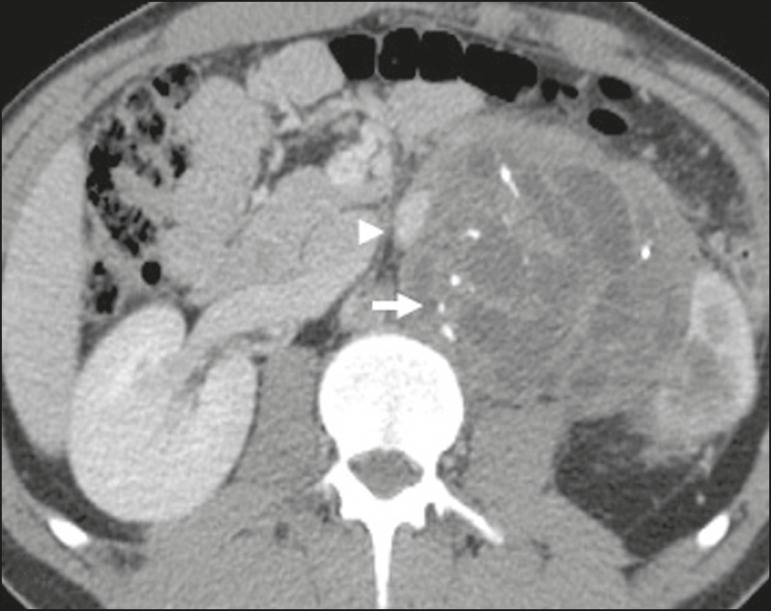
Mature teratoma in a 23-year-old female. CT scan showing a
